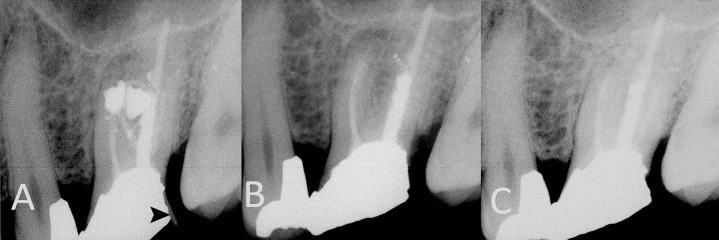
A) Periapical radiograph of a maxillary molar with radiographic masses of amalgam, the black arrowhead shows the path of gutta-percha used for finding the course of sinus tract (case No. 12); B) Immediately after endodontic surgery; C) the tooth 2 years after surgery
